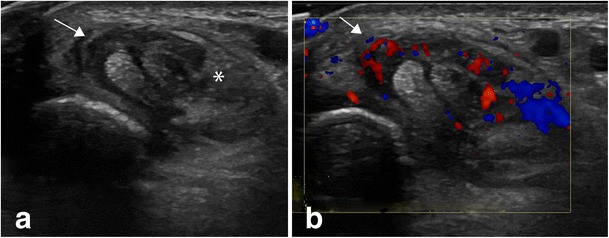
Ultrasound shows signs of tenosynovitis in the tibialis posterior and flexor digitorum longus tendons with hypertrophic synovium in the tendon sheet (a). The hypertrophic synovium can be both hypoechoic (arrow) and hyperechoic (asterisk). Hypervascularity is seen on colour Doppler examination (b)
